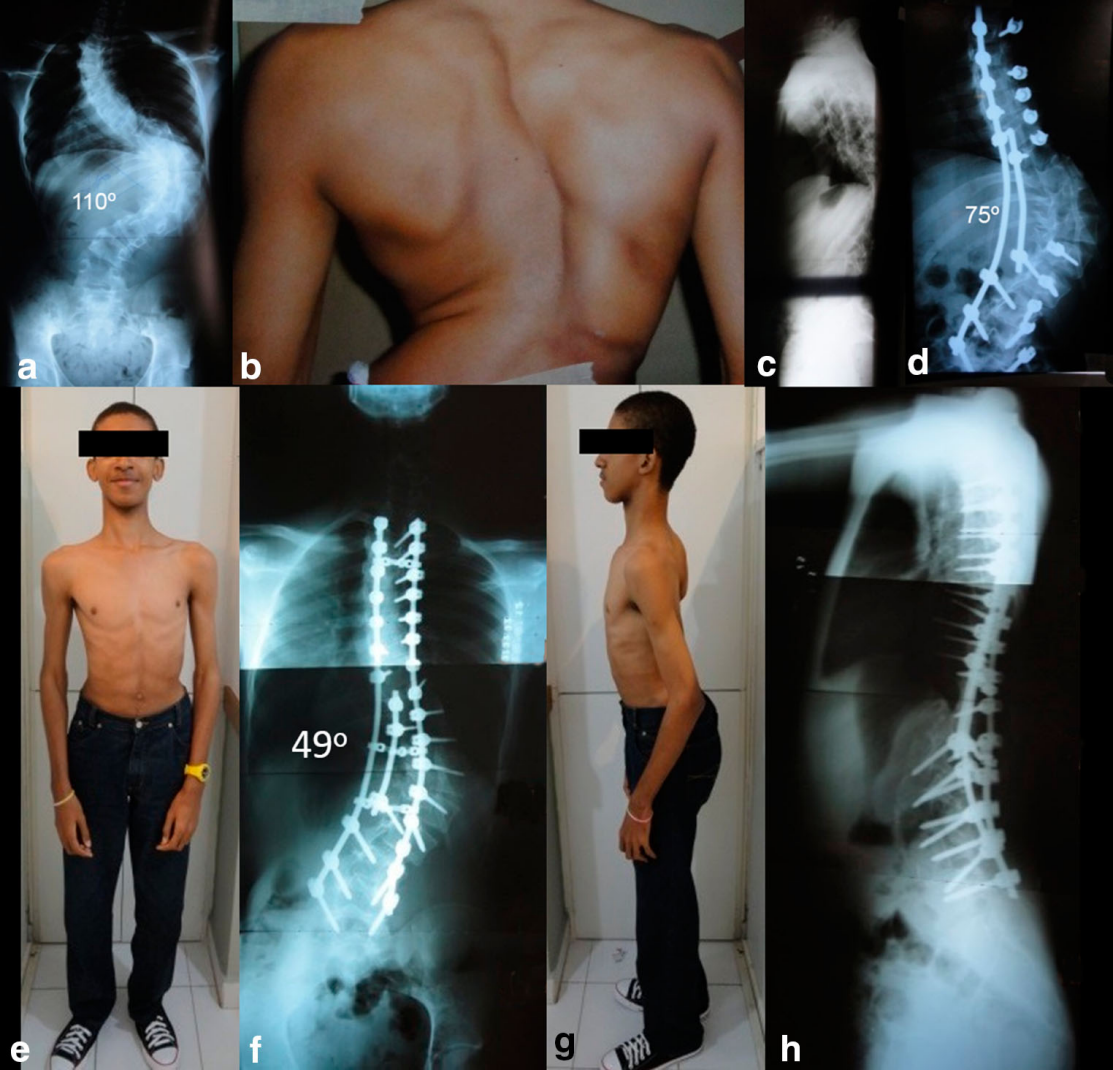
18-year-old male with severe rigid adolescent idiopathic scoliosis with 110° main thoracolumbar curve. Patient underwent a staged procedure. First, an internal distraction and posterior release followed by posterior spine fusion 1 week later. a–c Preoperative radiographical and clinical images. d PA radiograph after internal distraction. e–h Postoperative clinical and radiographical images
